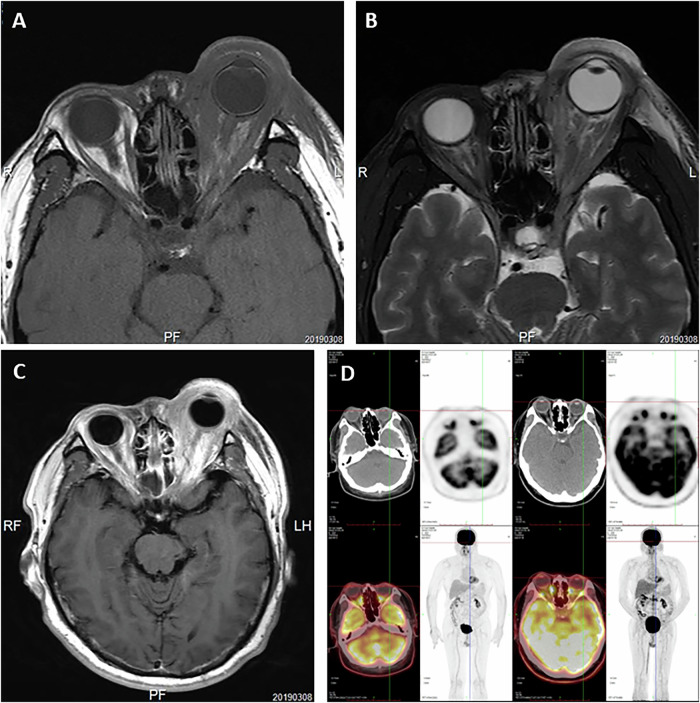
Ocular manifestations of ENKTL patients with ocular adnexal manifestations, case 3. A–C Orbital MRI: A poorly demarcated mass lesion is demonstrated in the left orbit involving the eyelids and periorbital tissues. The lesion exhibits an isointense signal on T1-weighted imaging and a hypointense signal on T2-weighted imaging, with marked contrast enhancement. The mass encases the globe with ill-defined margins between the lesion and both the optic nerve and extraocular muscles. D PET-CT: the left periorbital region shows patchy soft tissue density with mildly increased FDG uptake. The boundaries between the left extraocular muscles, optic nerve and surrounding soft tissues are indistinct. The orbital fat planes are obscured.
